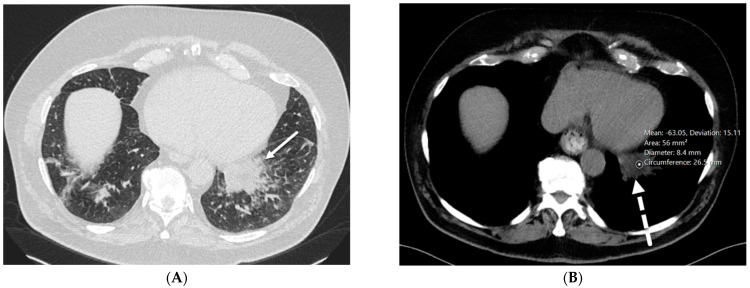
A 76-year-old male with chronic left lower lobe opacities due to lipoid pneumonia. The patient has a prior history of mineral oil ingestion for constipation. Axial chest CT images ((A) at baseline and (B) two years later) show interlobular septal thickening (solid white arrow) and fat attenuation (−40 to −60 HU) consolidation (dashed white arrow) in the left lower lobe without gross change.
